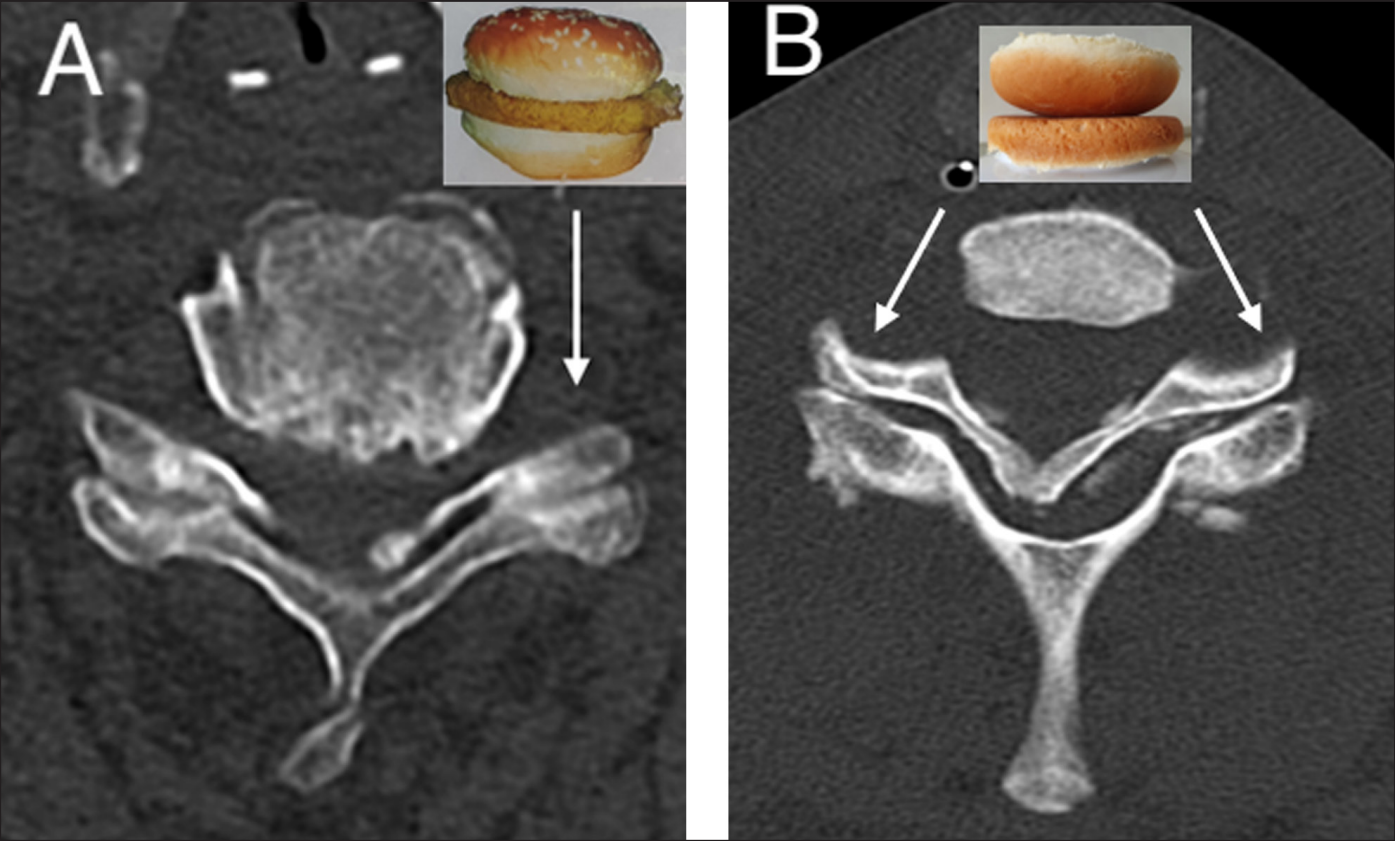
Hamburger bun sign, reverse hamburger bun sign and Naked facet sign. The “hamburger bun” sign resembles the normal appearance of facet joints on axial CT cervical spine scan of the before-mentioned 77-year-old man (A). Facet joint dislocations upset this relationship and reverse the orientation of the “bun” halves to each other. Hence, the “reverse hamburger bun sign”, also known as “naked facet sign”, represents the appearance on axial CT scan of an exposed facet joint at C6–7 level when it is dislocated (B). These signs are often seen in either unilateral or bilateral facet joint dislocation as a result of flexion-distraction injury—where the inferior articular facet of the upper vertebra projects anterior to the superior articular facet of the inferior vertebra.
